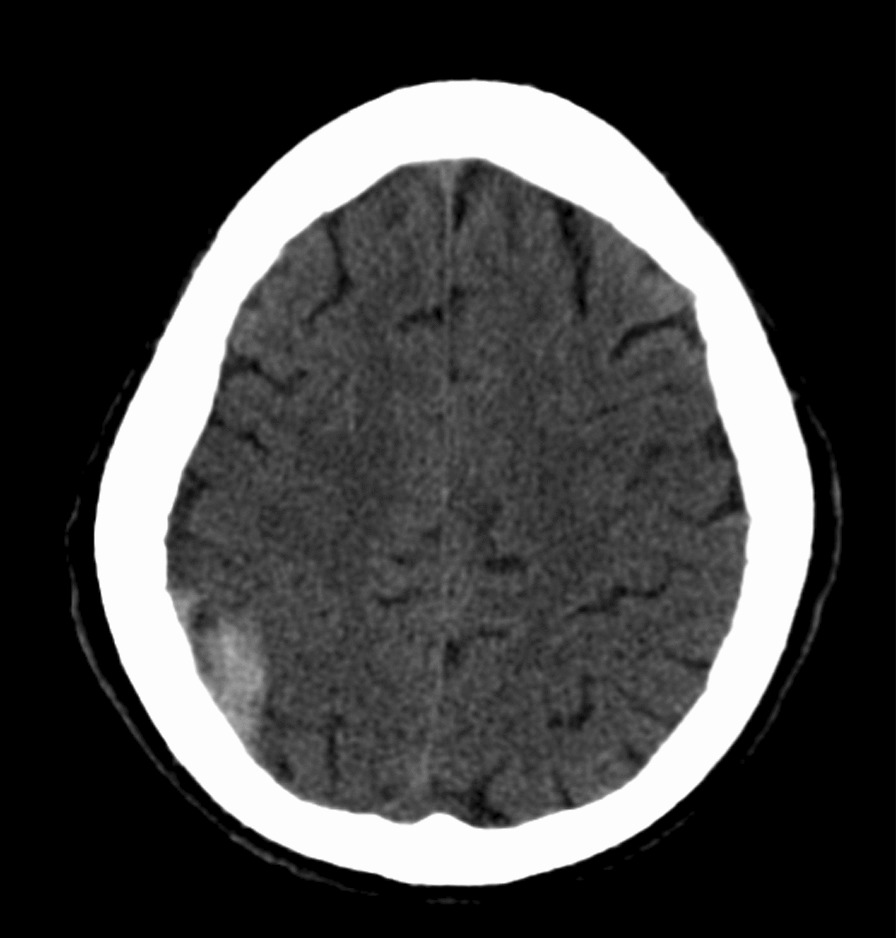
Computed tomography of the Head revealing a right posterior sub-acute epidural hematoma
